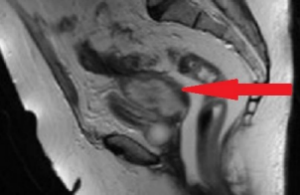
Magnetic resonance imaging (MRI) of the lower pelvis in the sagittal plane with intravenous contrast
